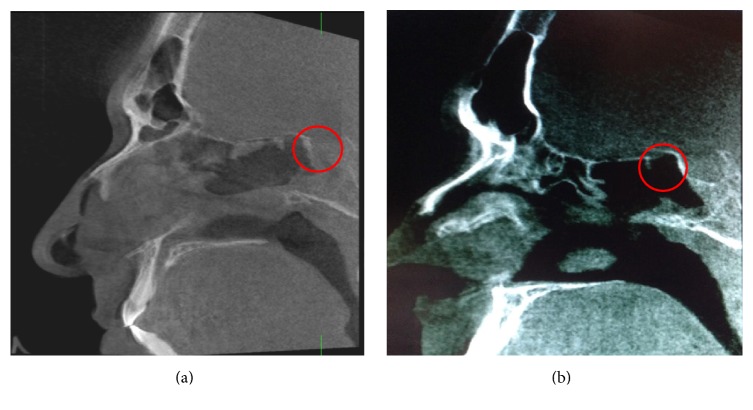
Case 5. (a) Preoperative CT scan showing a CSF leak in the anterior wall of the sella turcica. (b) Three-month postoperative CT showing a less dense bony closure of the surgical defect of the anterior wall of the sella turcica.
